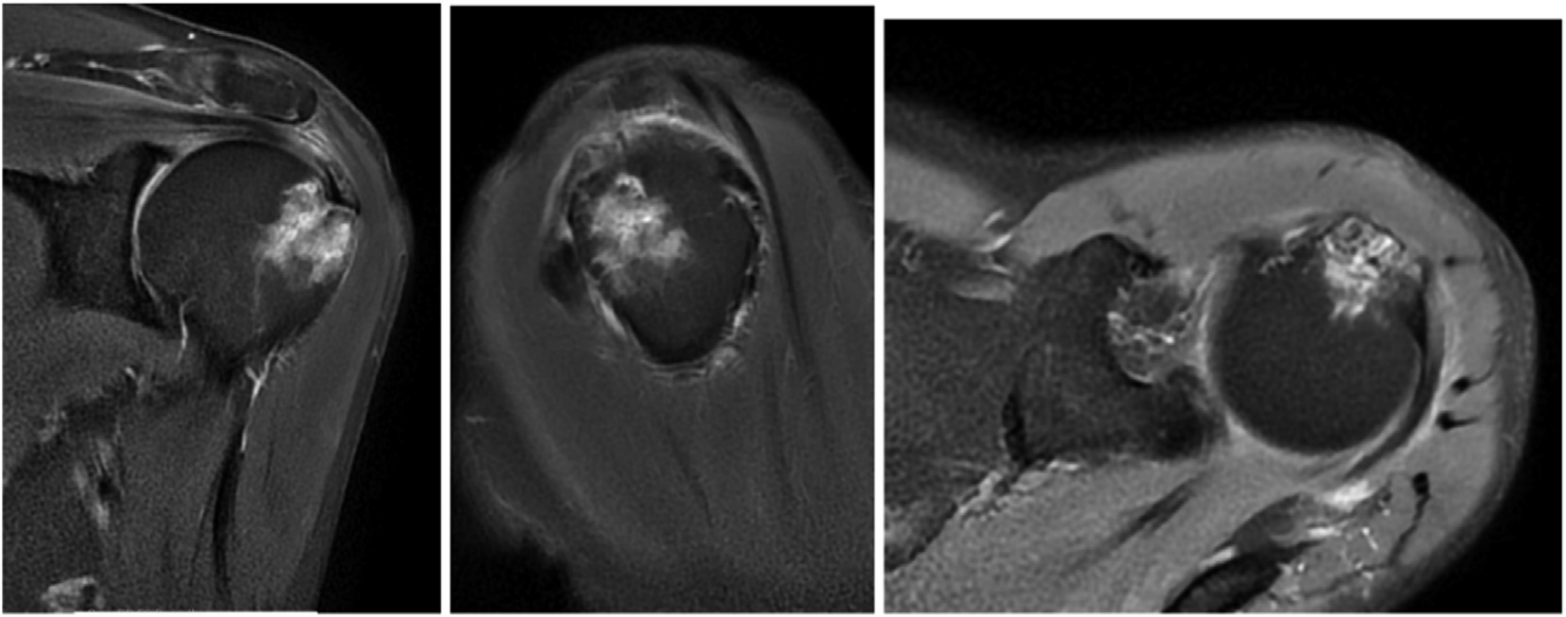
MRI with RhoFatsat sequences shows a significant intraosseous edema at the footprint of the supra tendinous tendon at the greater tubercule with irregularity and loss of cortical continuity. *MRI*, magnetic resonance imaging.
